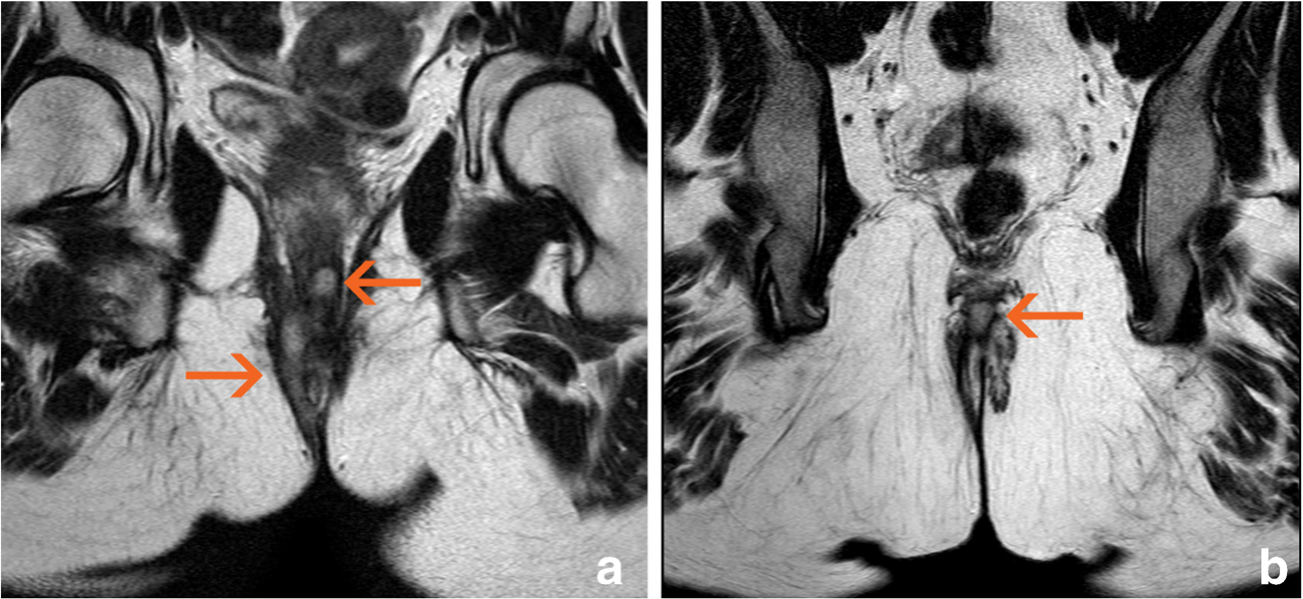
Partial response after anti-TNF therapy. a Axial T2-weighted sequences at baseline show a complex bilateral trans-sphincteric fistula with posterior “horseshoe” fluid. Hyperintense paths are present both near the external opening and at the intersphincteric level (arrows). b Axial T2-weighted sequences after 24 weeks of treatment reveal that the distal part of the fistula shows signs of healing (hypointensity); however, the intersphincteric fluid collection persists (arrow)
